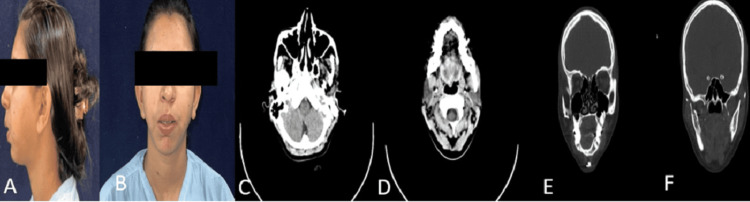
(A and B) Extra-oral profile (2023); (C and D) CT scan; (E and F) CT scan (coronal cuts)
